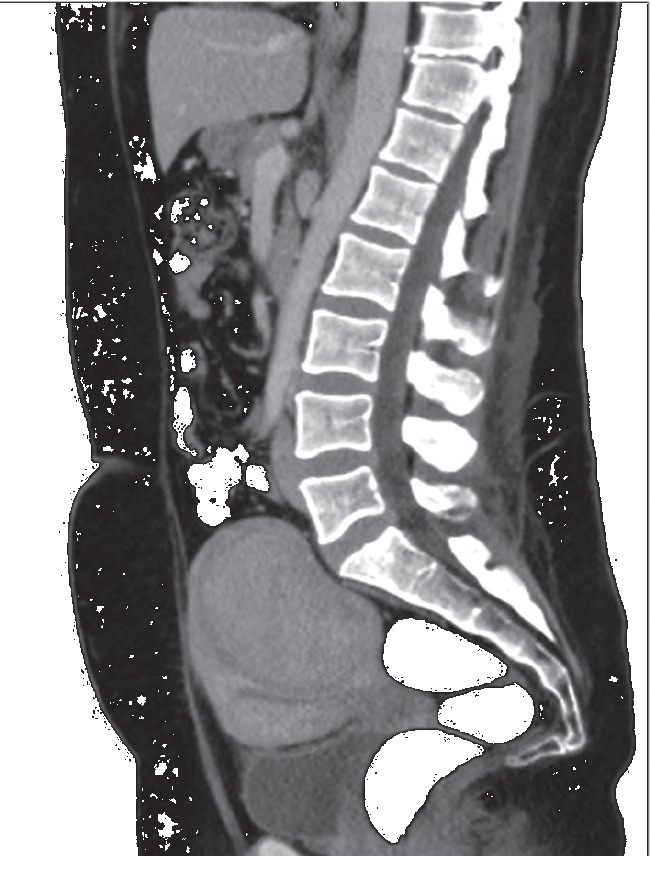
CT scan of abdomen (sagittal plane) showing the intramural uterine fibroid.
